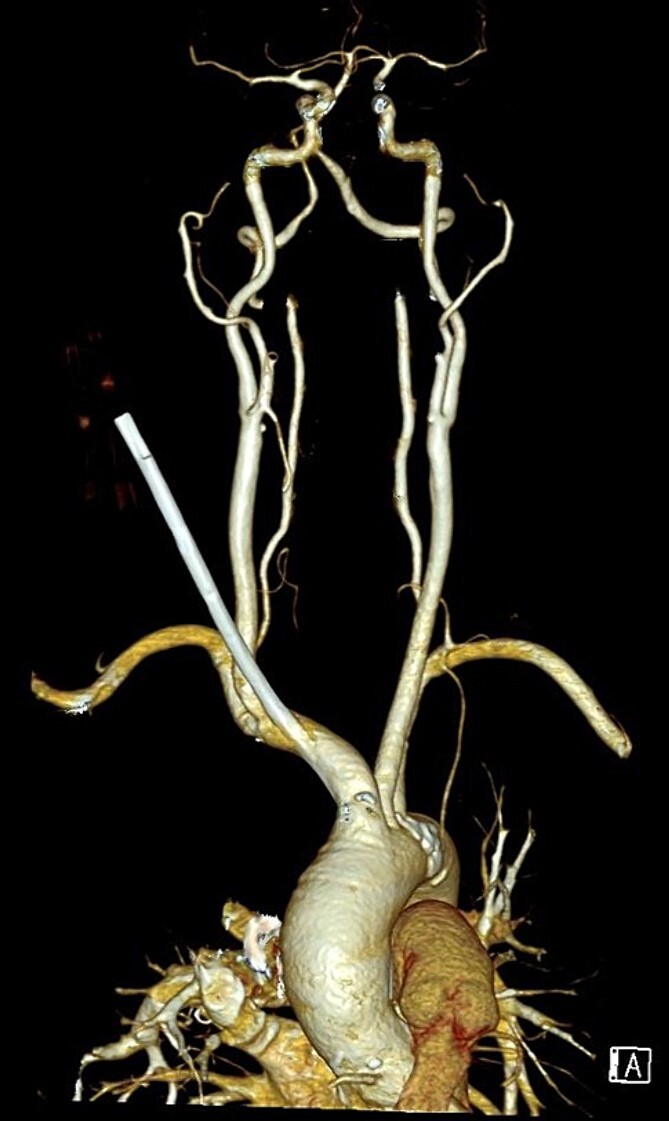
3-dimensional reconstruction of a CT angiogram of the neck and thorax depicting the ectopic haemodialysis catheter in the brachiocephalic artery.
